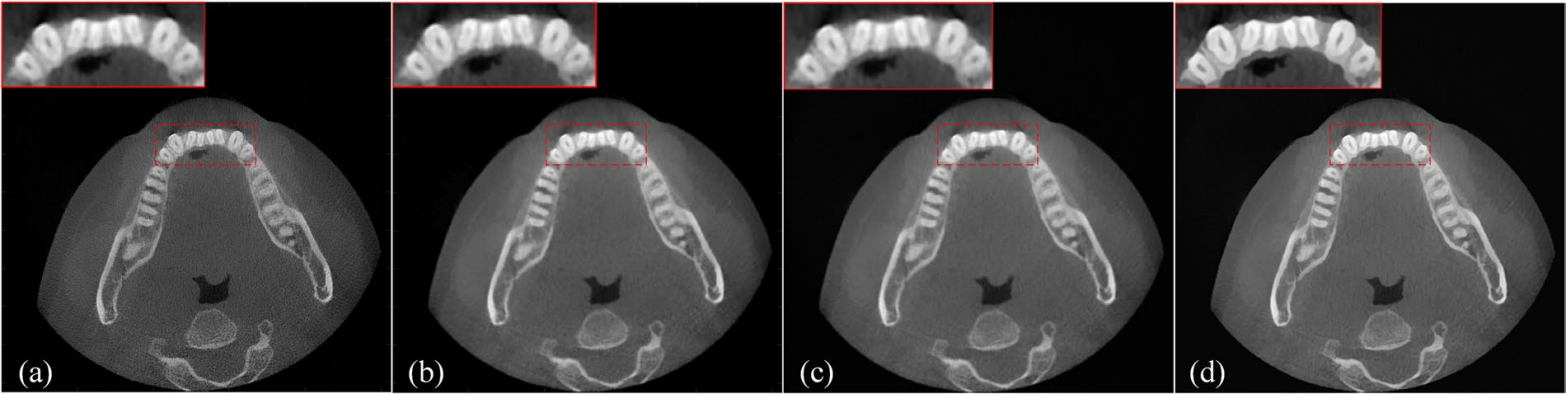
Comparison of restored images for one downsampled slice of the dental CT images. (a) Reference full-sampling CT image reconstructed using the ART-TV method with 360 projections. (b) Input LR CT image reconstructed using the ART-TV method with 120 projections. (c) Restored image using the bicubic interpolation method. (d) Image reconstructed using the proposed method with 4 iterations. All images are displayed in the same window. These images are all in 16-bit format, and the display window is [0, 10000].
